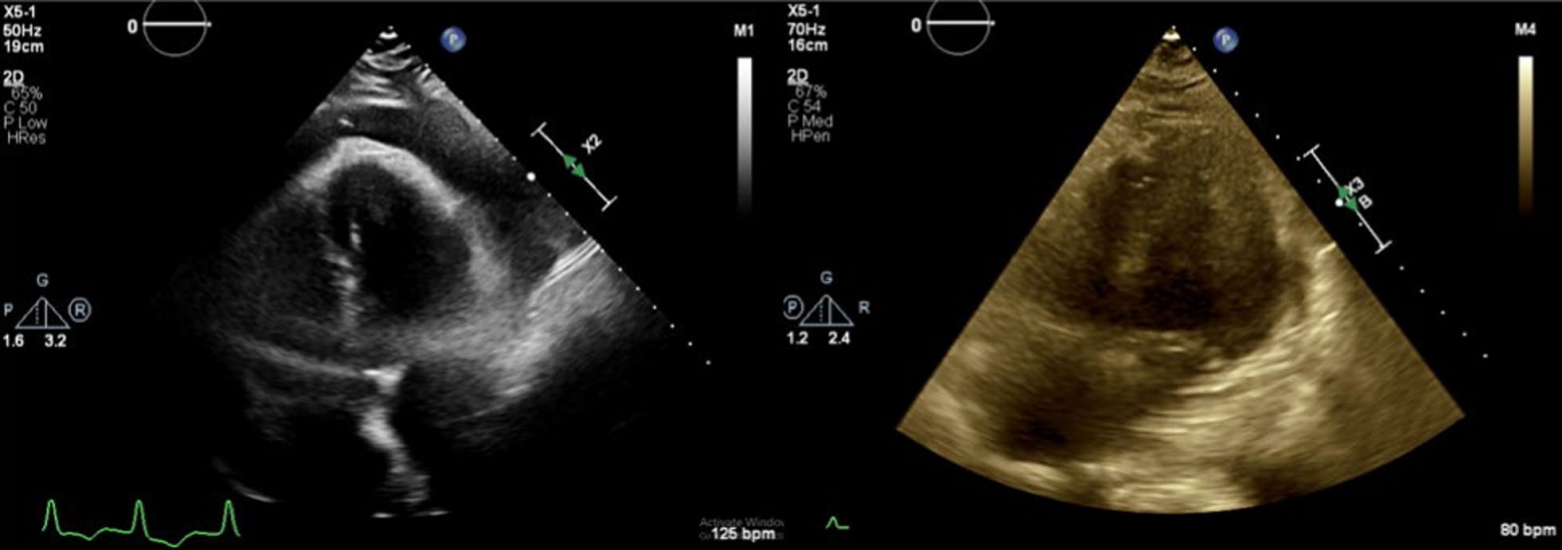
Case 2; TTE apical four-chamber view before (left image) and after (right image) pericardiocentesis
